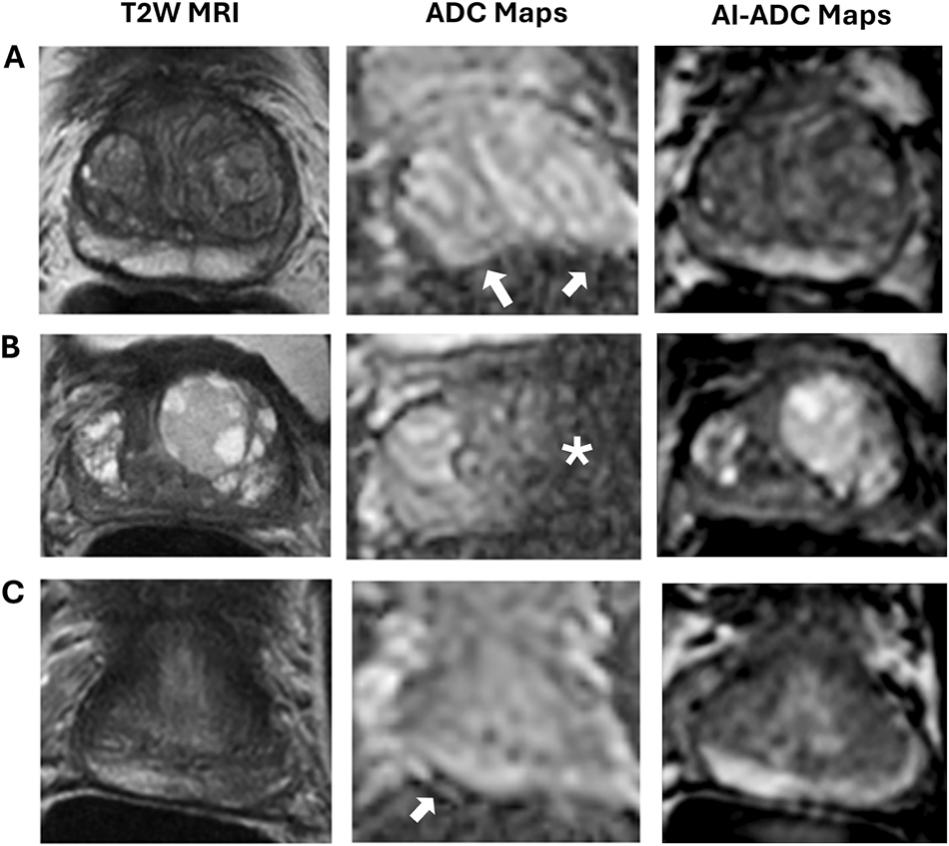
Examples of three patients with T2W MRI, ADC maps, and AI-ADC maps. An 80-year-old patient with a serum PSA of 10.3 ng/mL presented with a standard ADC map distorted due to rectal gas-associated susceptibility artifacts (arrows) with a concave boundary artifact in the posterior prostate; however, the AI-ADC map generated from T2W MRI showed no artifact (A). An 83-year-old patient with serum PSA of 1.8 ng/mL had a standard ADC map with significant noise in the left hemi-prostate secondary to a left hip prosthesis (asterix), but the AI-ADC map did not demonstrate this noise (B). A 57-year-old patient with serum PSA of 0.7 ng/mL had a standard ADC map affected by rectal gas susceptibility artifacts (arrow), with the corresponding AI-ADC map remaining artifact-free (C). All readers gave higher quality scores for AI-ADC maps for these three patients
